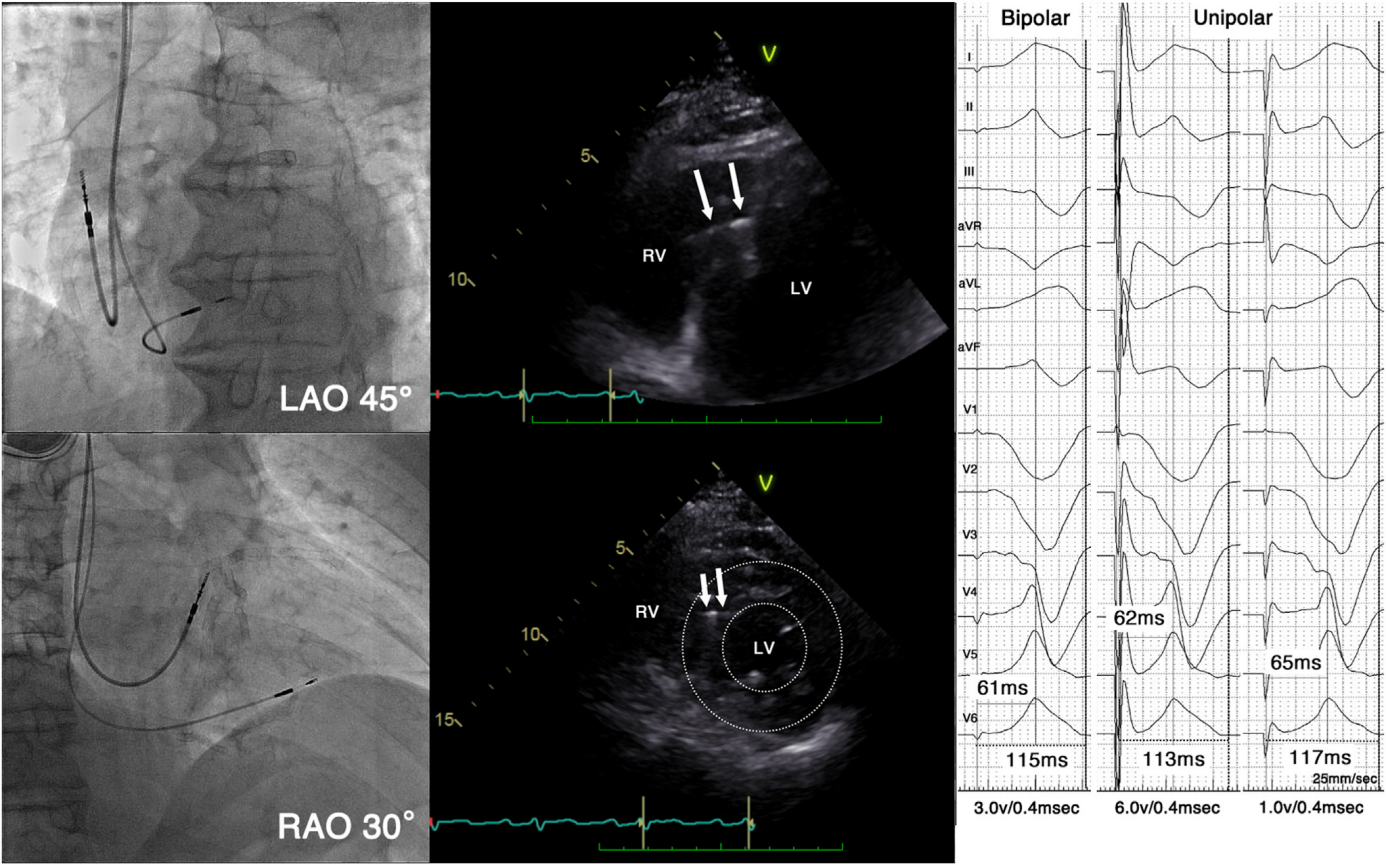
Fluoroscopy and transthoracic echocardiography images in right anterior oblique (RAO) and left anterior oblique (LAO) views showing the pacing lead location after the pacemaker reimplantation. The transthoracic echocardiographic images showed that the ventricular lead was not completely perpendicular to the septal myocardium but had advanced anteriorly and toward the apex (arrow). The R-wave peak time (RWPT) in lead V_6_ was 61 ms with a bipolar voltage of 3.0 V with a 0.4 ms pulse width, which was almost the same value as that before the lead extraction. The RWPT increased rather than decreased as the pacing stimulus amplitude decreased from 6 V to 1 V. LV = left ventricle; RV = right ventricle; V = volt.
